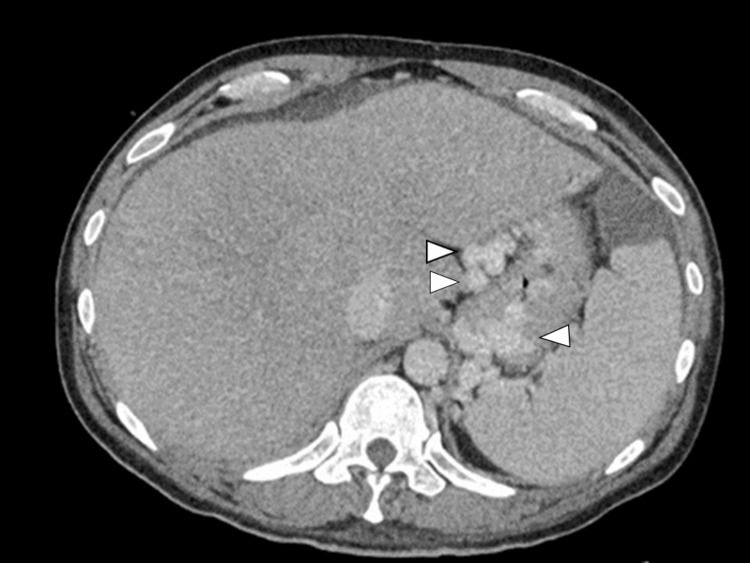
The contrast-enhanced CT scan indicates esophagogastric varices, marked by the arrowhead.
